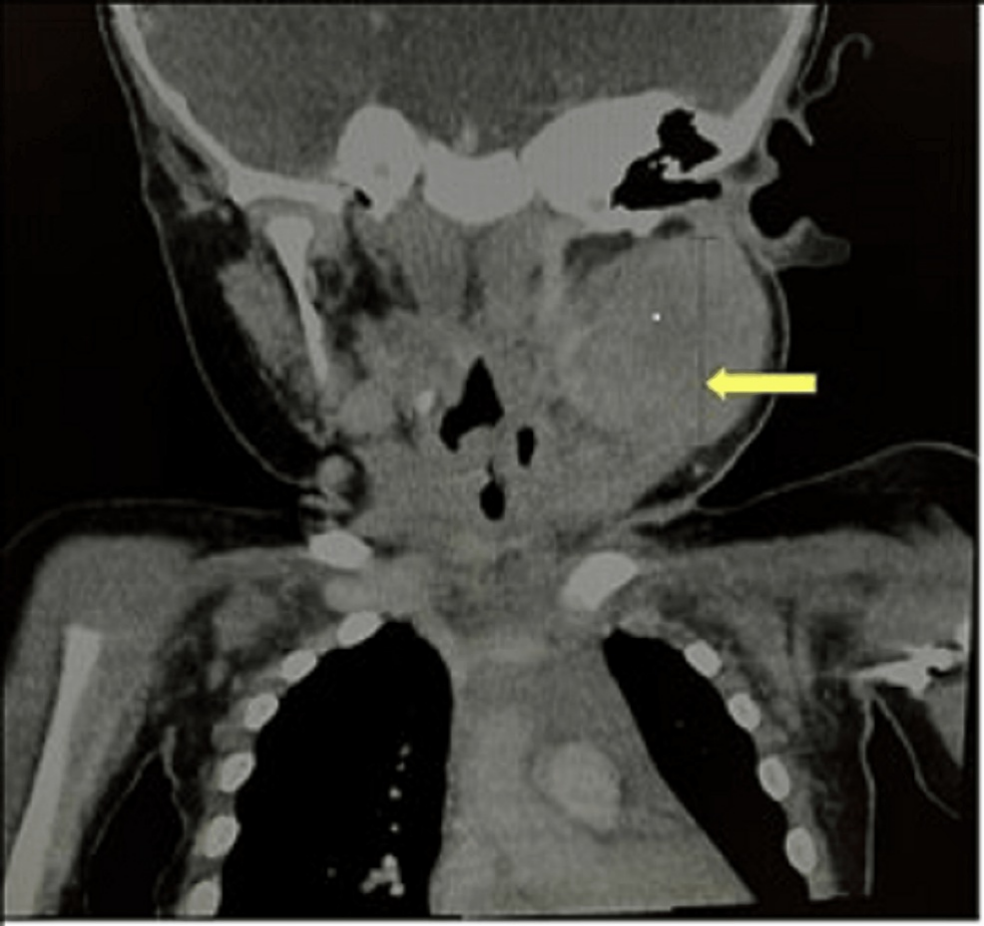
CT with contrast coronal section showing a 3.5 cm area of decreased attenuation on the left anterior cervical area (pointed by yellow arrow)
